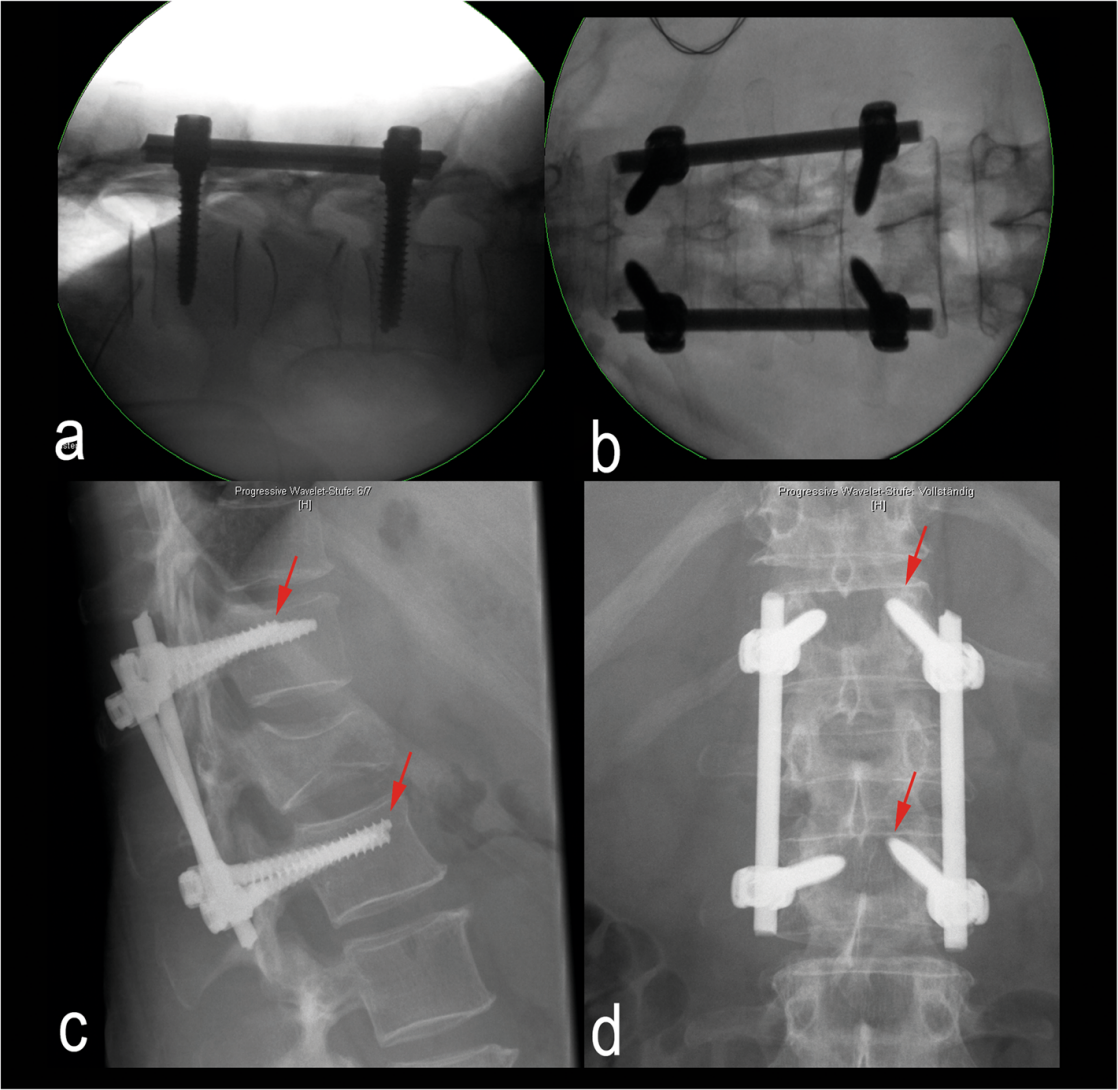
Intraoperative radiographs in prone position in lateral view (a) and ap view (b). Early implant failure 4 days after surgery in standing radiograph lateral view (c) and ap view (d) showing a combined movement (toggling) (red arrows)
